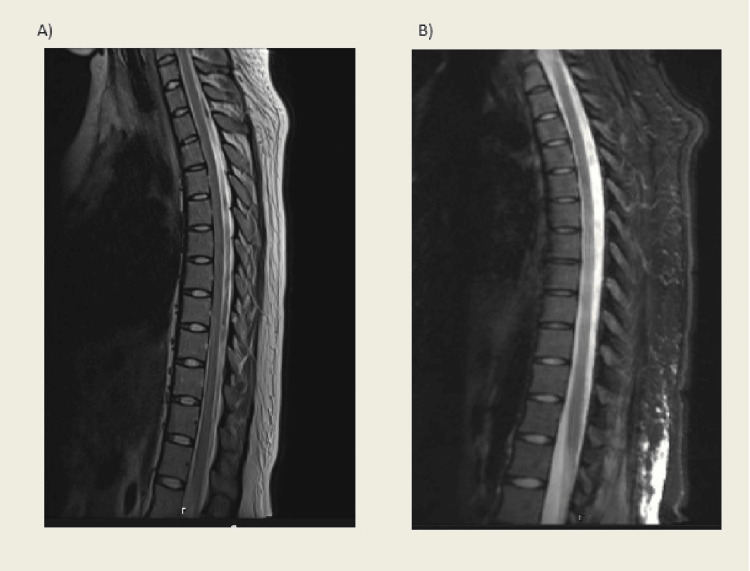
T2 weighted MRI of thoracic spine without spinal cord abnormality. A represents MRI completed on post-op day #1 and B represents the MRI completed on post-op day #21. Both are normal without any obvious etiology of paralysis.
